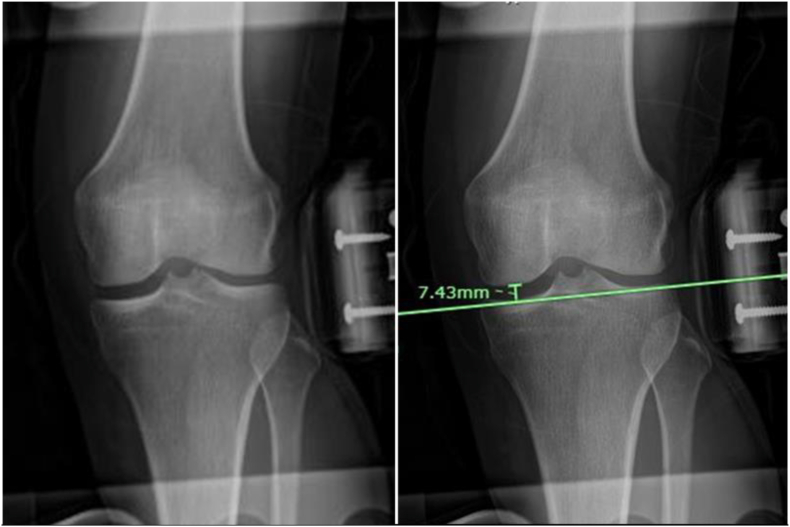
(a) Valgus stress radiograph in AP view of left the knee. (b) Radiographic measurement of the medial joint space width; drawn line connecting the subchondral bone of the medial and lateral tibial condyles. From this line, draw a vertical perpendicular line to the most distal point of the medial femoral condyle. The distance of this vertical line was recorded as the medial joint space width.
